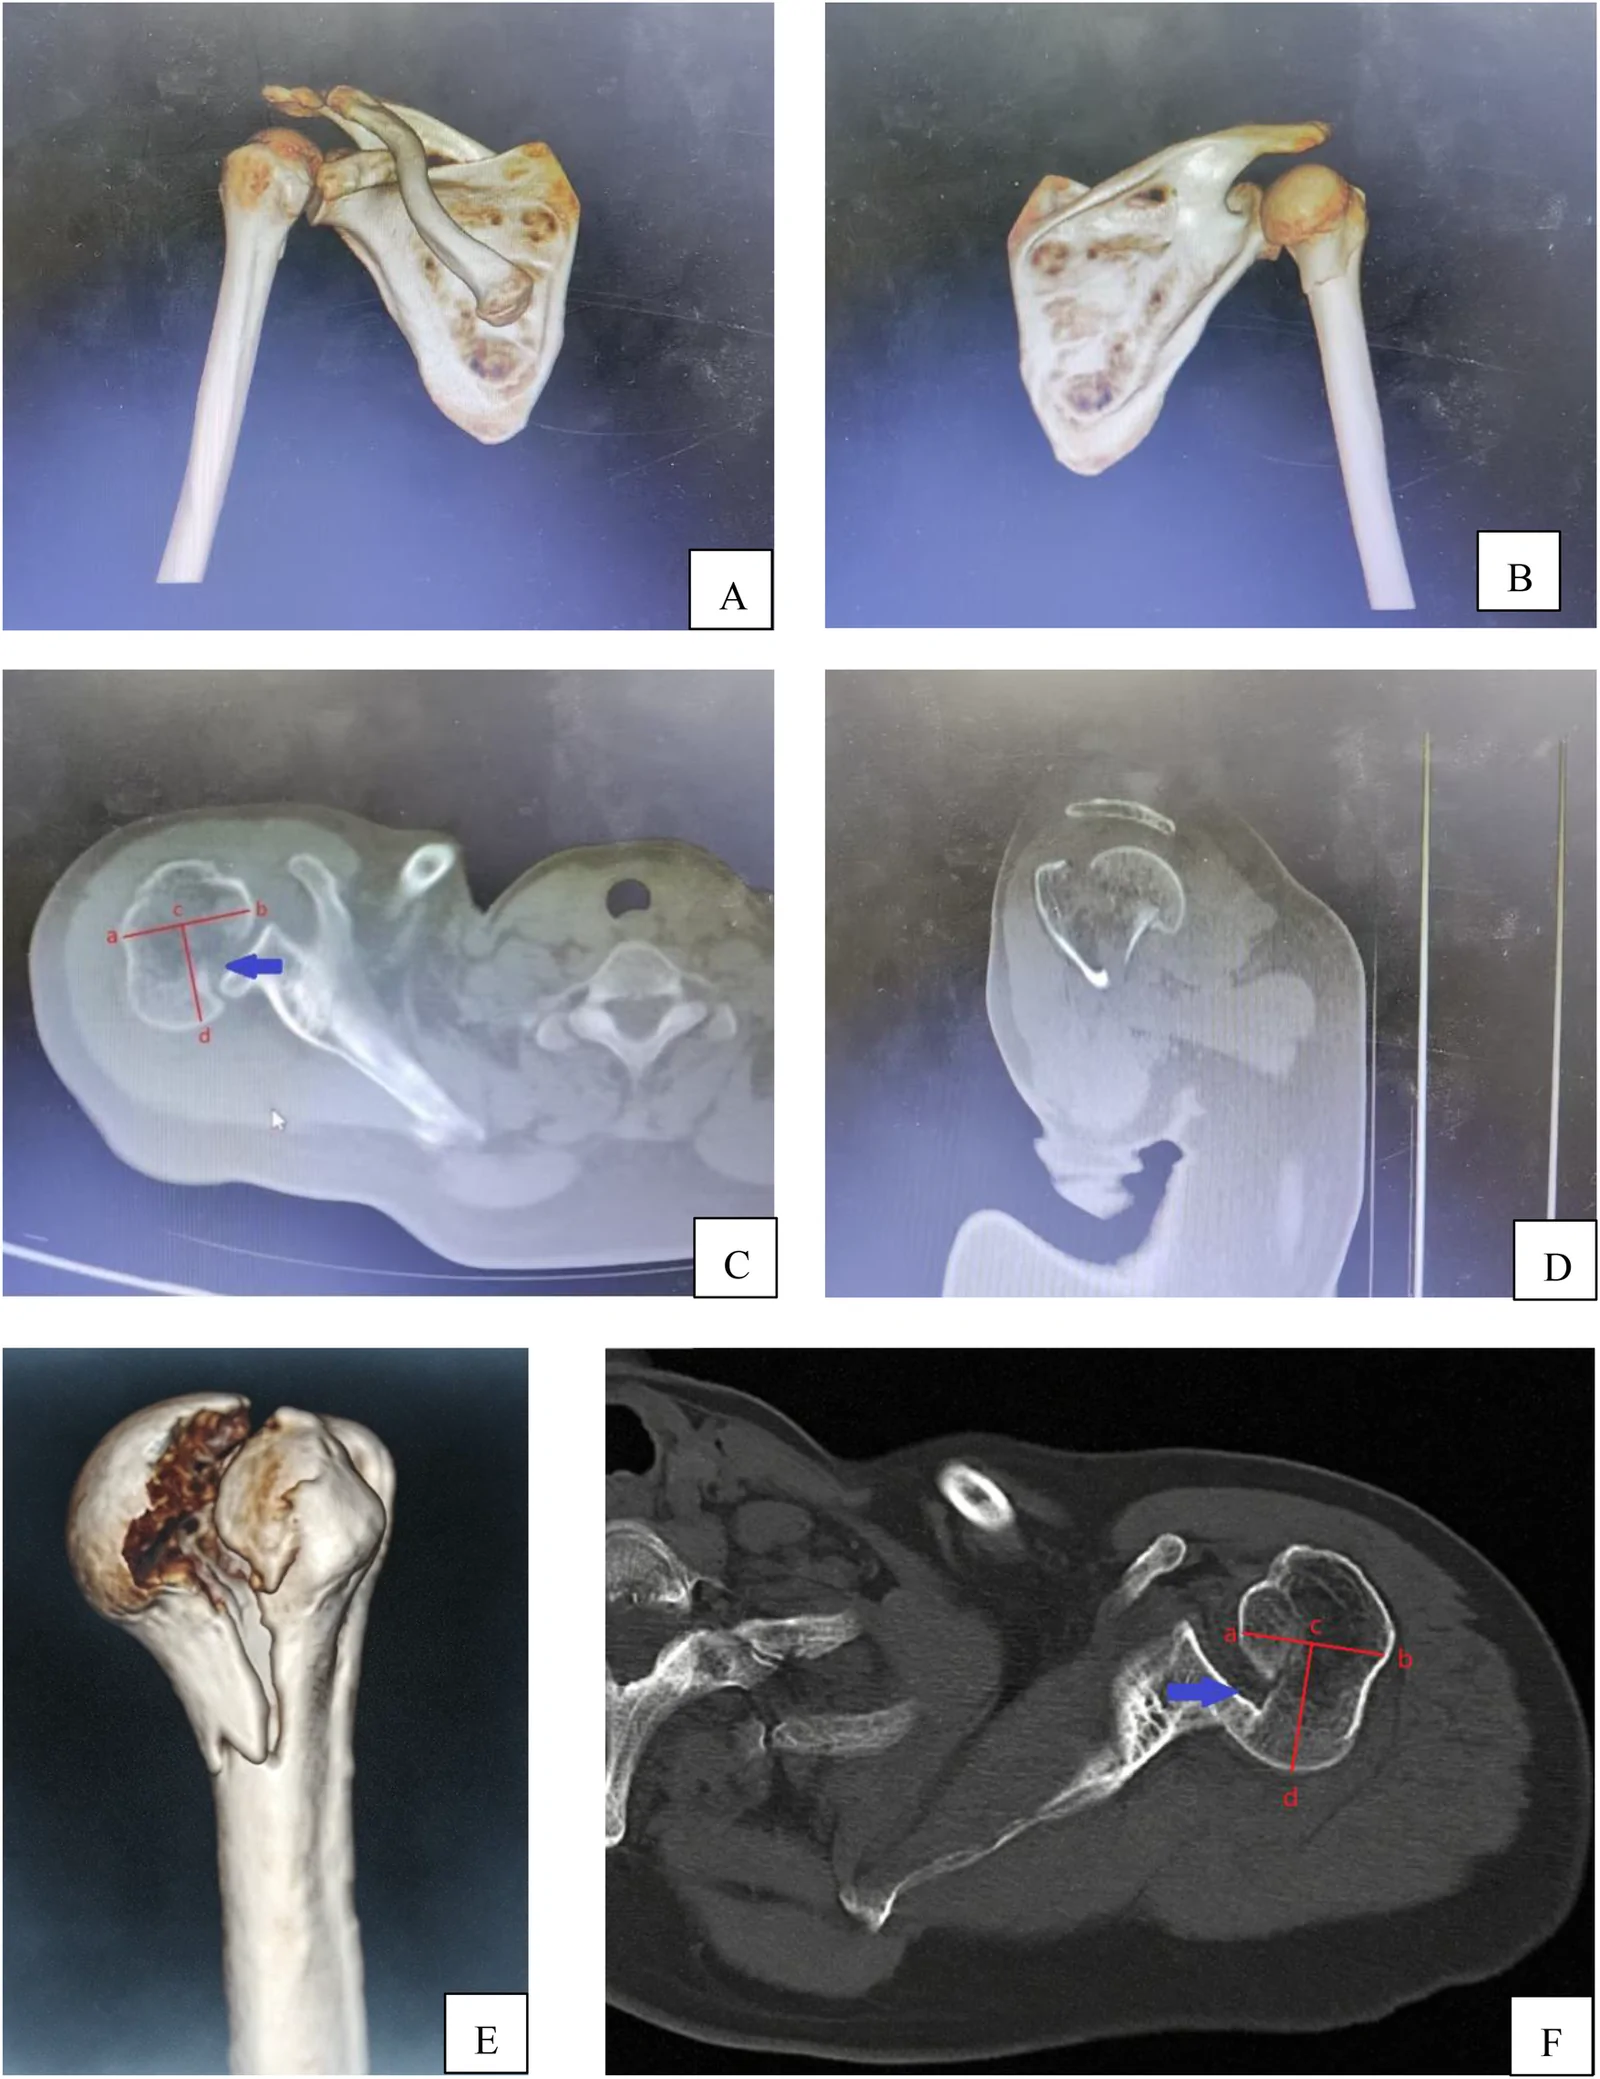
Preoperative 3D CT of the patient NO.4: (A) Anterior view. (B) Posterior view. (C) Axial view. Line ab is the articular surface connecting line, and line cd bisects the articular surface. The blue arrow indicates the reverse Hill-Sachs lesion. The defect area is between 25% and 50%. (D) Sagittal view. Preoperative 3D CT of the patient NO.7: (E) Anterior view. (F) Axial view.
